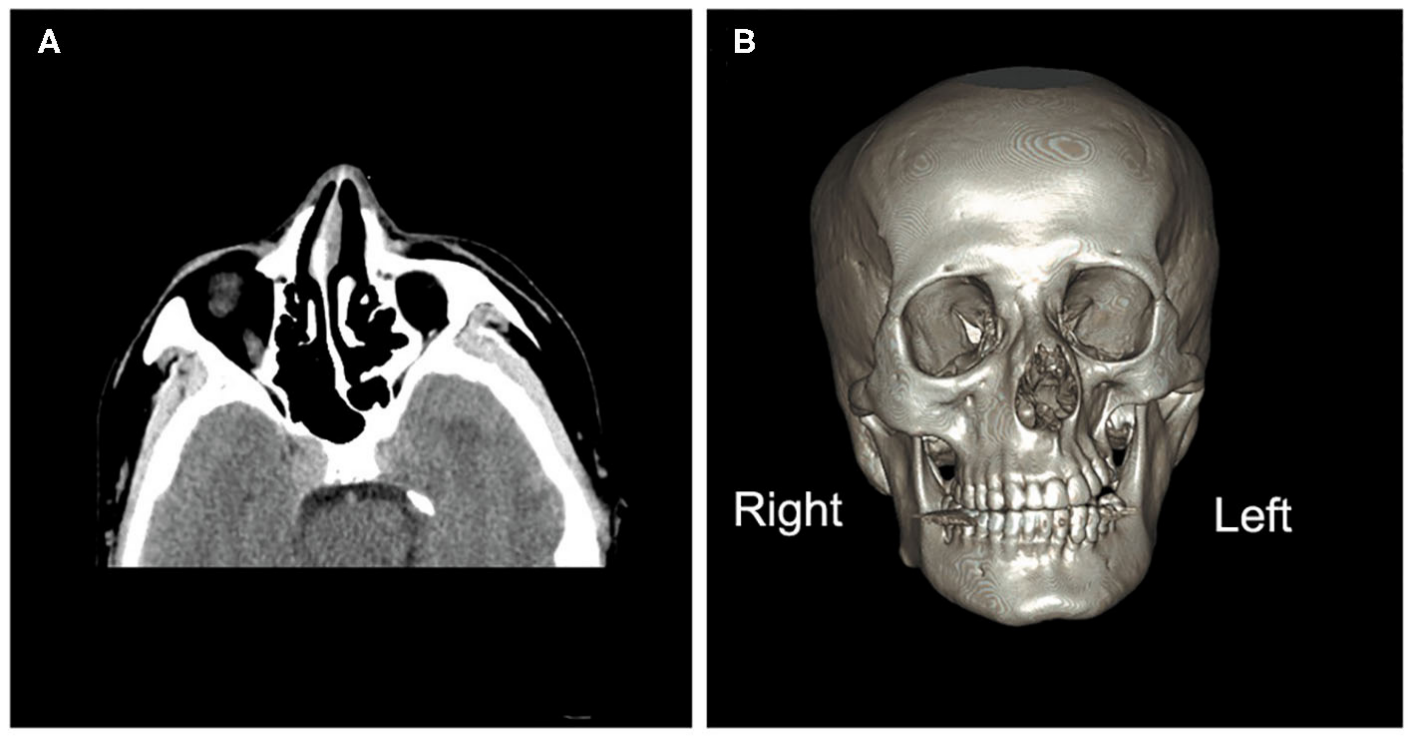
Brain computed tomography images. (A) Brain computed tomography (axial image) depicting under-development of the bone structure on the left side of the face compared with the right, including the nasal bone, nasal septum, sphenoid bones, and ethmoid bones. (B) A three-dimensional image of the brain depicting atrophy of the orbit, zygomatic bone, and teeth on the left side.
